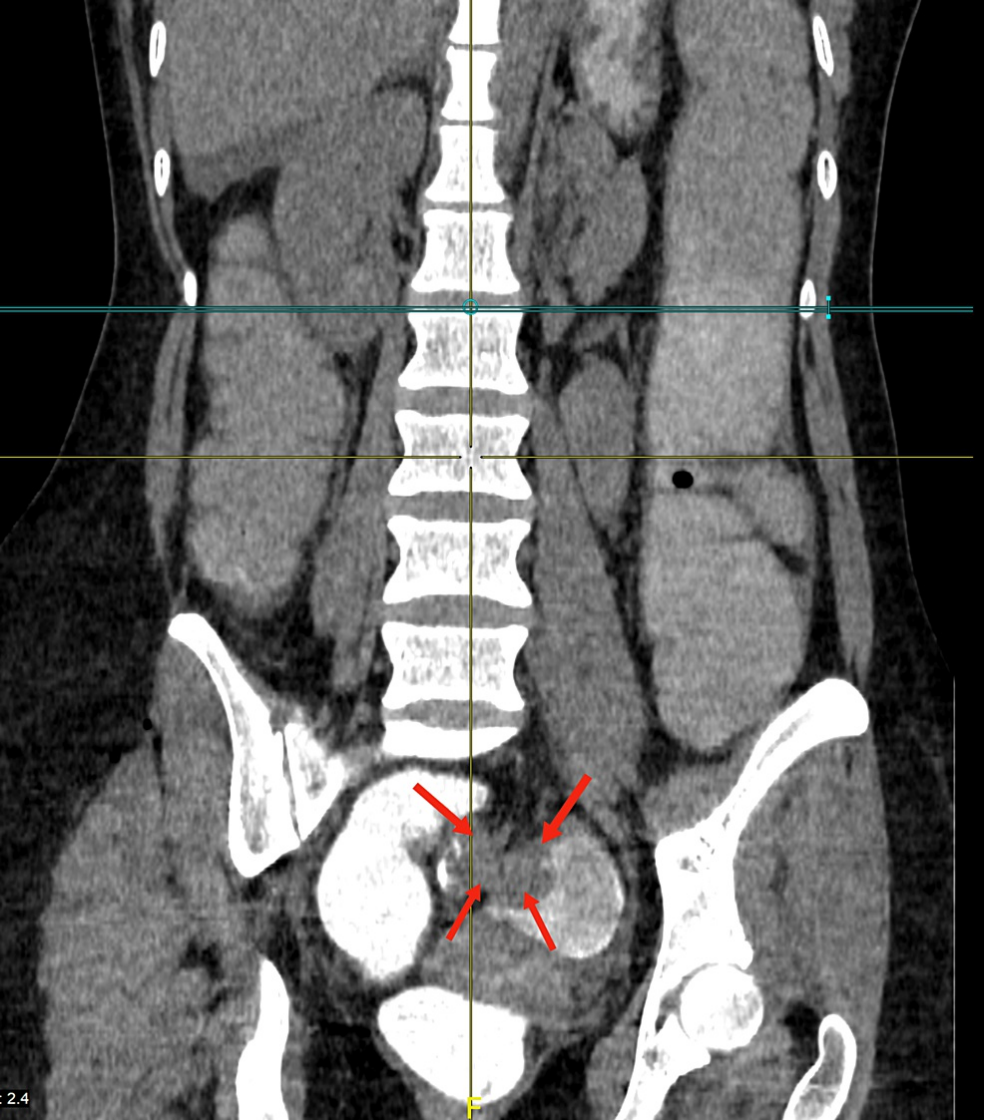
Abdominal CT scan with rectal contrast showing a filling rectum with a minimal trickling of contrast proximal to an obstructive mass (red arrows). CT, computed tomography
